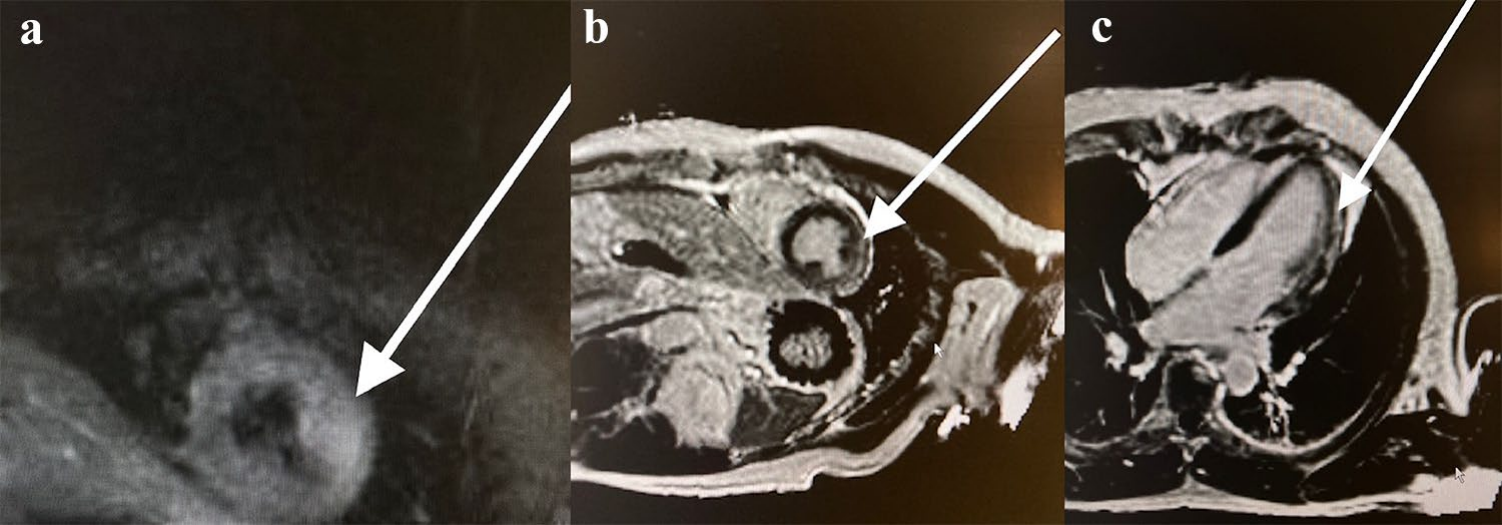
a Cardiac MRI, T2 sequence, arrow indicating focal edema anterolateral medial (criterion 1 in ESC definition).  b Short-axis view showing subepicardial gadolinium late enhancement anterolateral medial (criterion 3 in ESC definition [4]). c Corresponding four-chamber view
